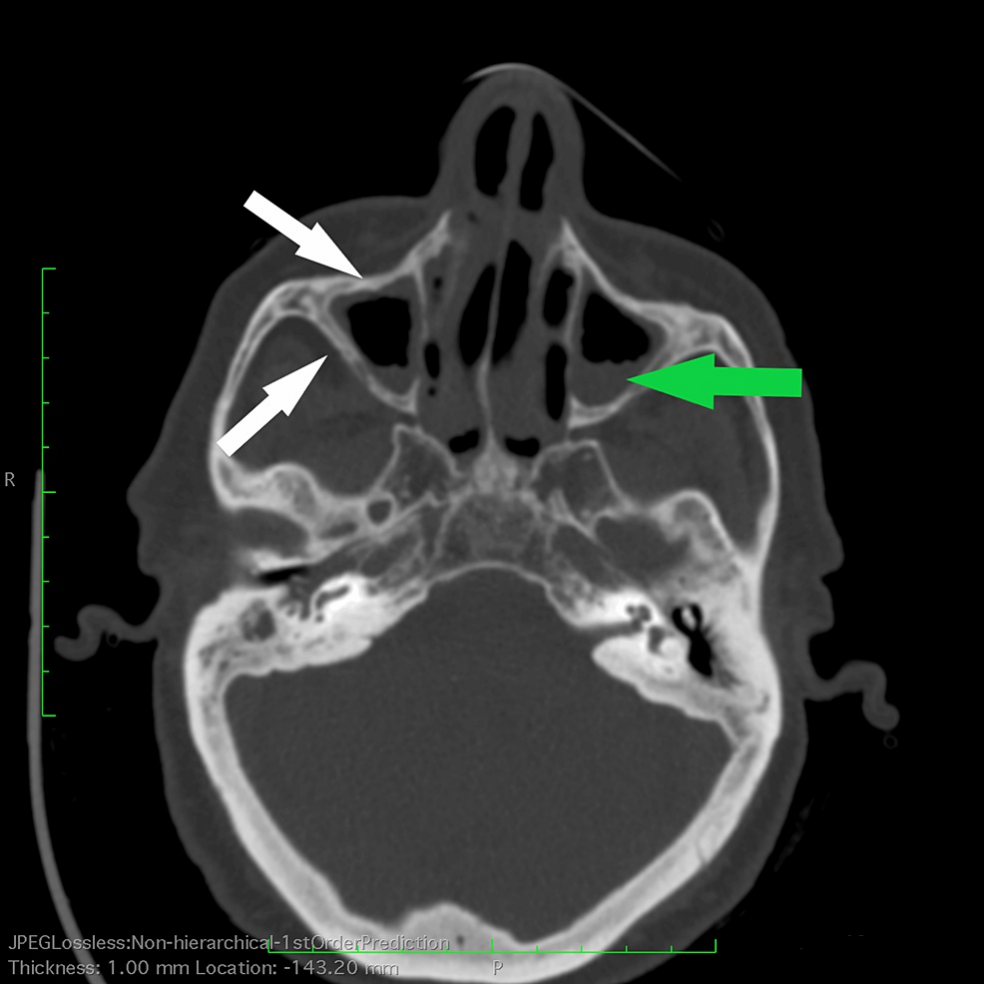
Sinus CT scan. Sinus CT scan (bone window) showing secretions and mucosal thickening (green arrow) in both maxillary sinuses as well as sclerotic thickened bone involving the sinus walls (white arrows), suggestive of acute and chronic sinusitis.
